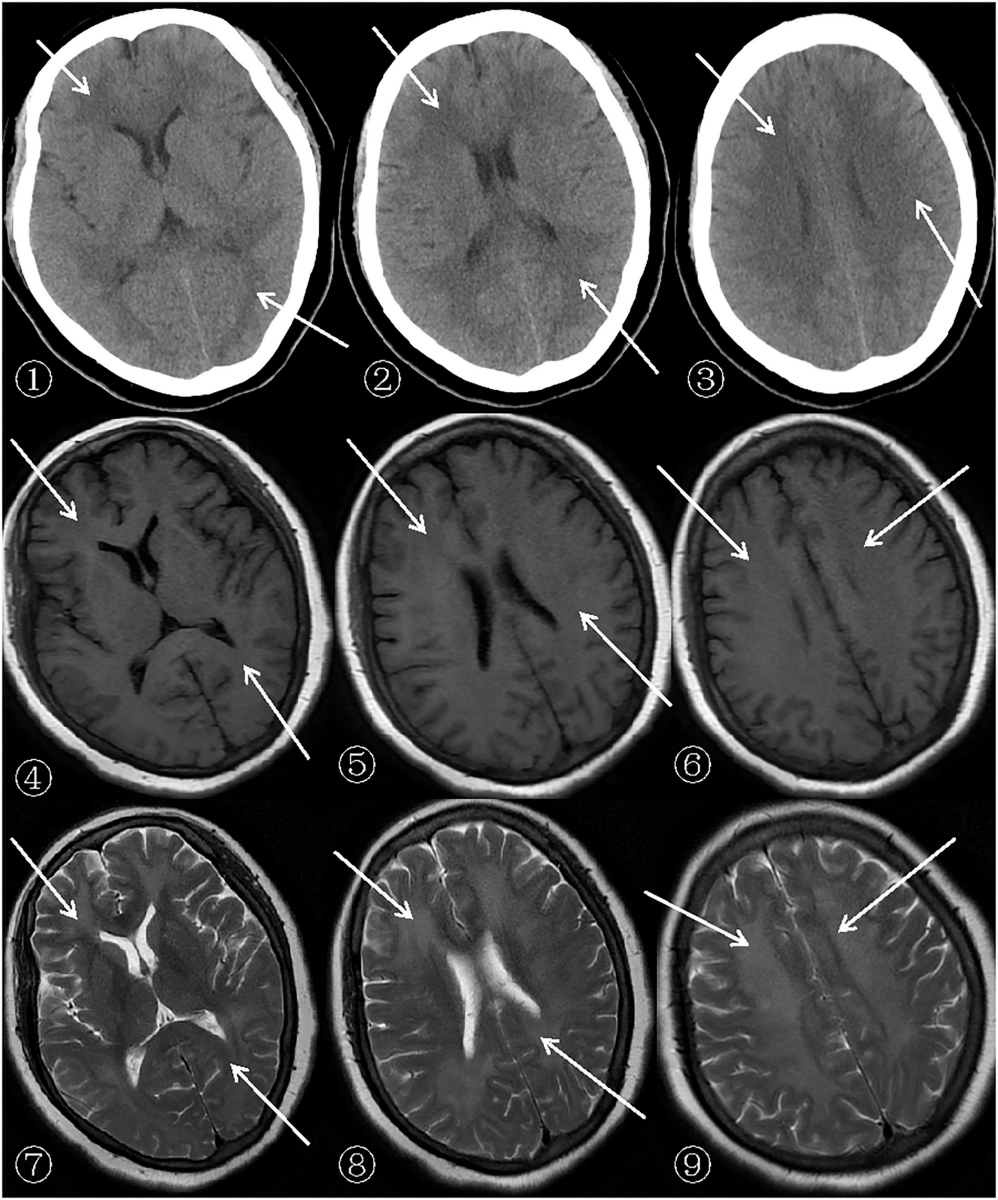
Patient's cerebral CT (General Electric Company, 64-slice Lightspeed CT) and MRI (SIEMENSAGFWB, skyra 3.0 tesla) scans.
